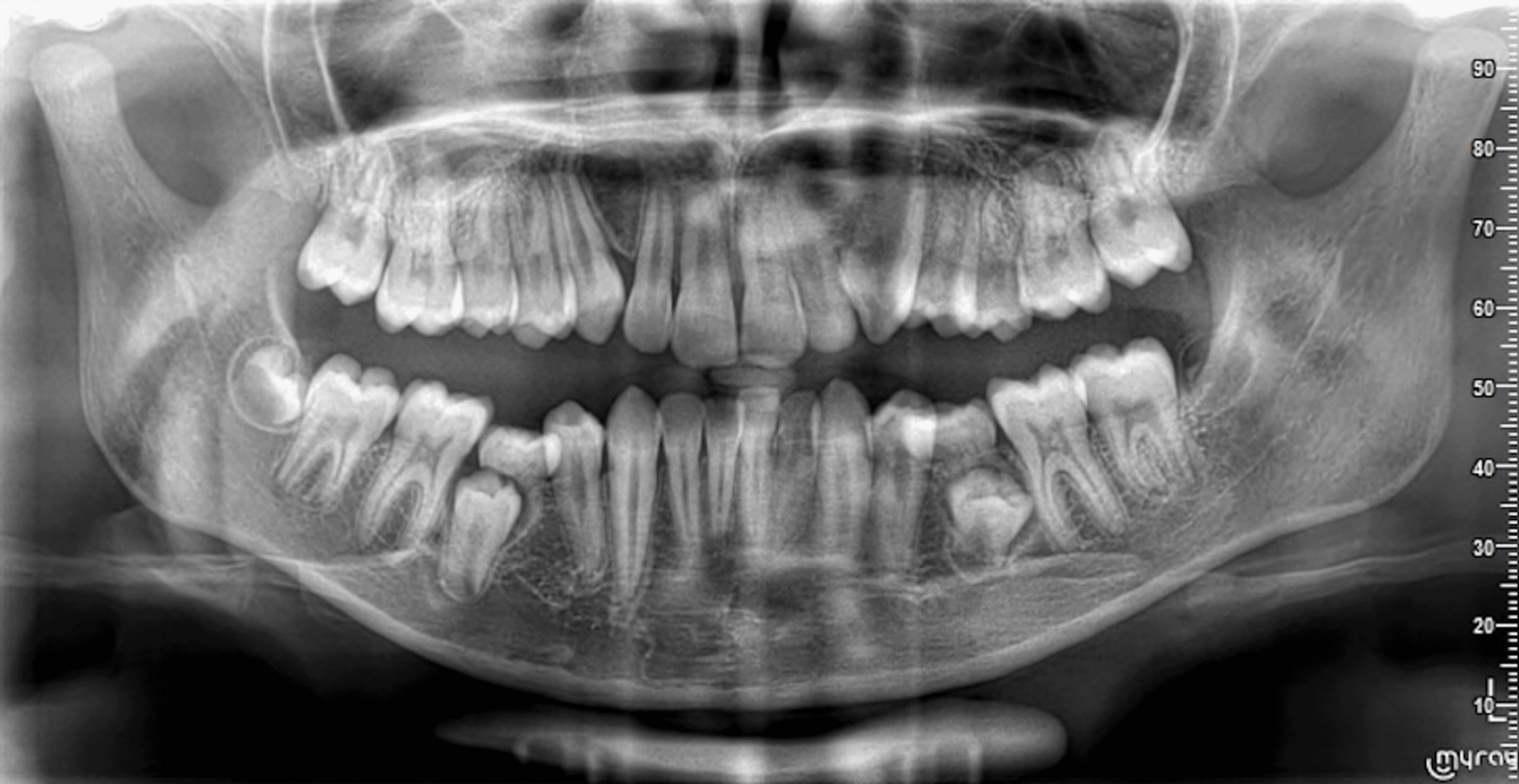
Normal panoramic radiographic appearance
